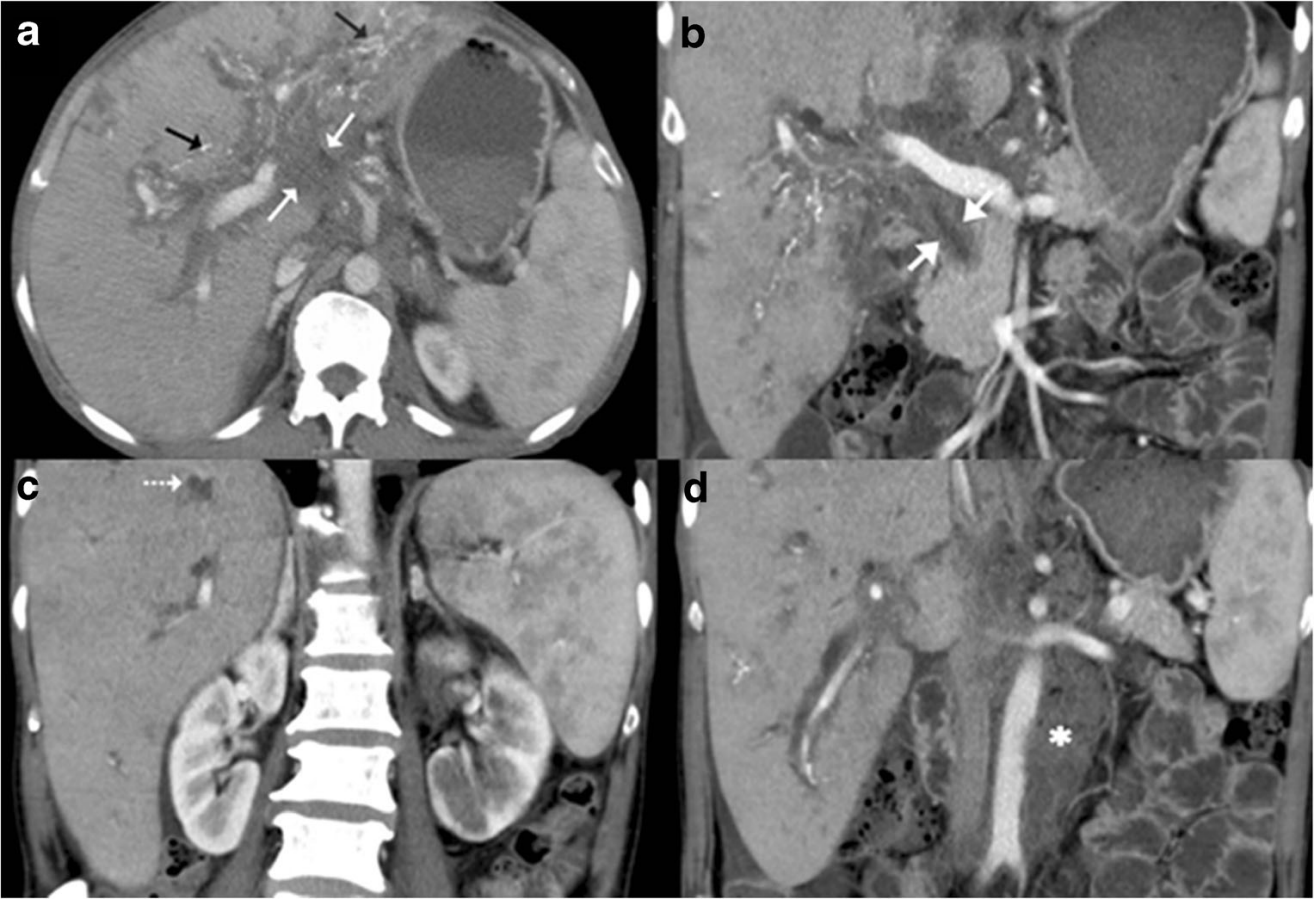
a, b Contrast-enhanced CT shows an ill-defined mass in the periportal location (*short, thin arrows*) with associated rather marked thickening of the extrahepatic common duct (*short, thick arrow*). Extensive calcifications can be seen along the biliary radicals (*black arrows*). c Concurrent hypodense liver lesions either granulomas or cholangitic abscesses (*dotted arrows*). d Associated large retroperitoneal nodal mass (*asterisk*) is visualised
